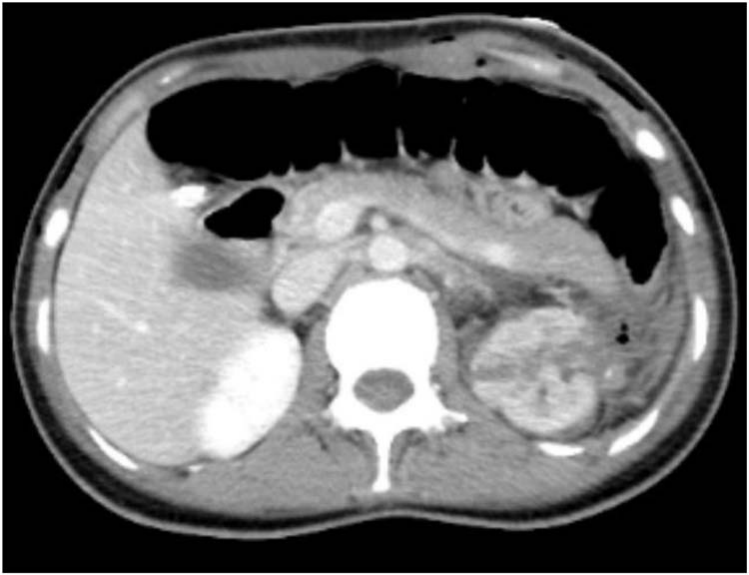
CT shows uneven density and disordered structure in the upper pole of the left kidney, with turbidity and increased density in the surrounding fat spaces.
